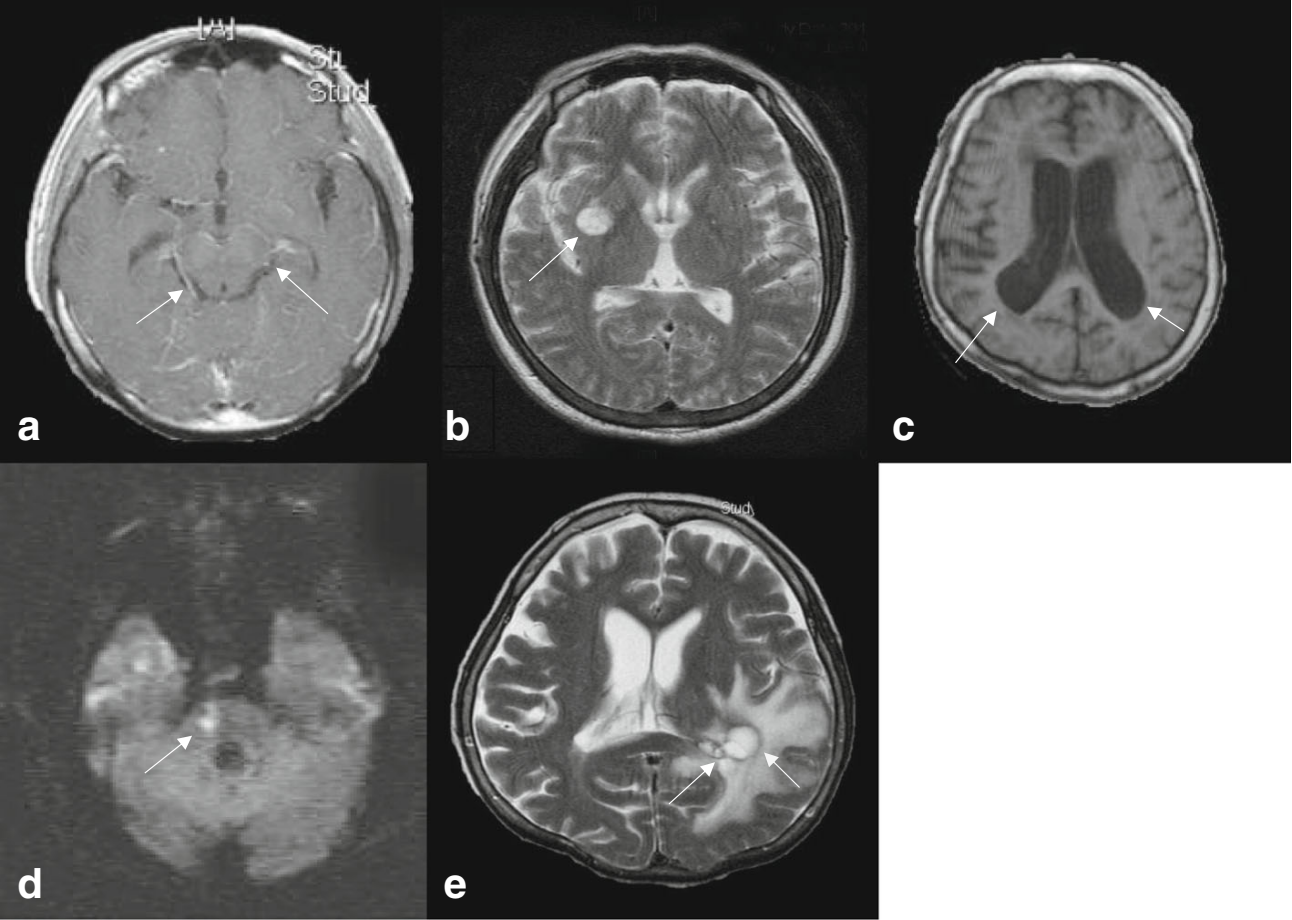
The main magnetic resonance imaging features of the elderly patients with cryptococcal meningitis. a: Gadolinium contrast-enhanced T1-weighted image (T1WI) showing basal meningeal enhancement (arrow). b: T2-weighted magnetic resonance image (T2WI) showing pseudocysts (arrow). c: T1WI showing ventricular dilatation. d: Diffusion-weighted image showing a recent cerebral infarct at the right pons (arrow). e: T2WI showing cryptococcoma (arrow)
